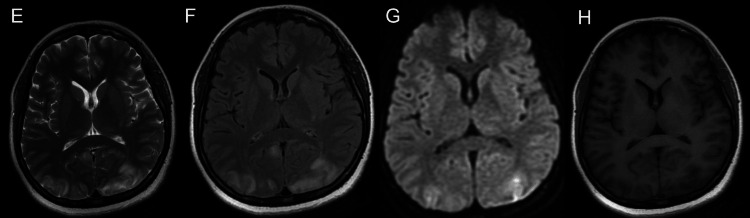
MRI at the level of internal capsule. Altered signal intensity which appears hyperintense on T2WI (E), FLAIR (F); T2 shine through in DWI (G); hypointense on T1WI (H) involving bilateral parieto-occipital regions. DWI - Diffusion-weighted imaging FLAIR - Fluid attenuated inversion recovery MRI - Magnetic resonance imaging T1WI - T1 weighted imaging T2WI - T2 weighted imaging
